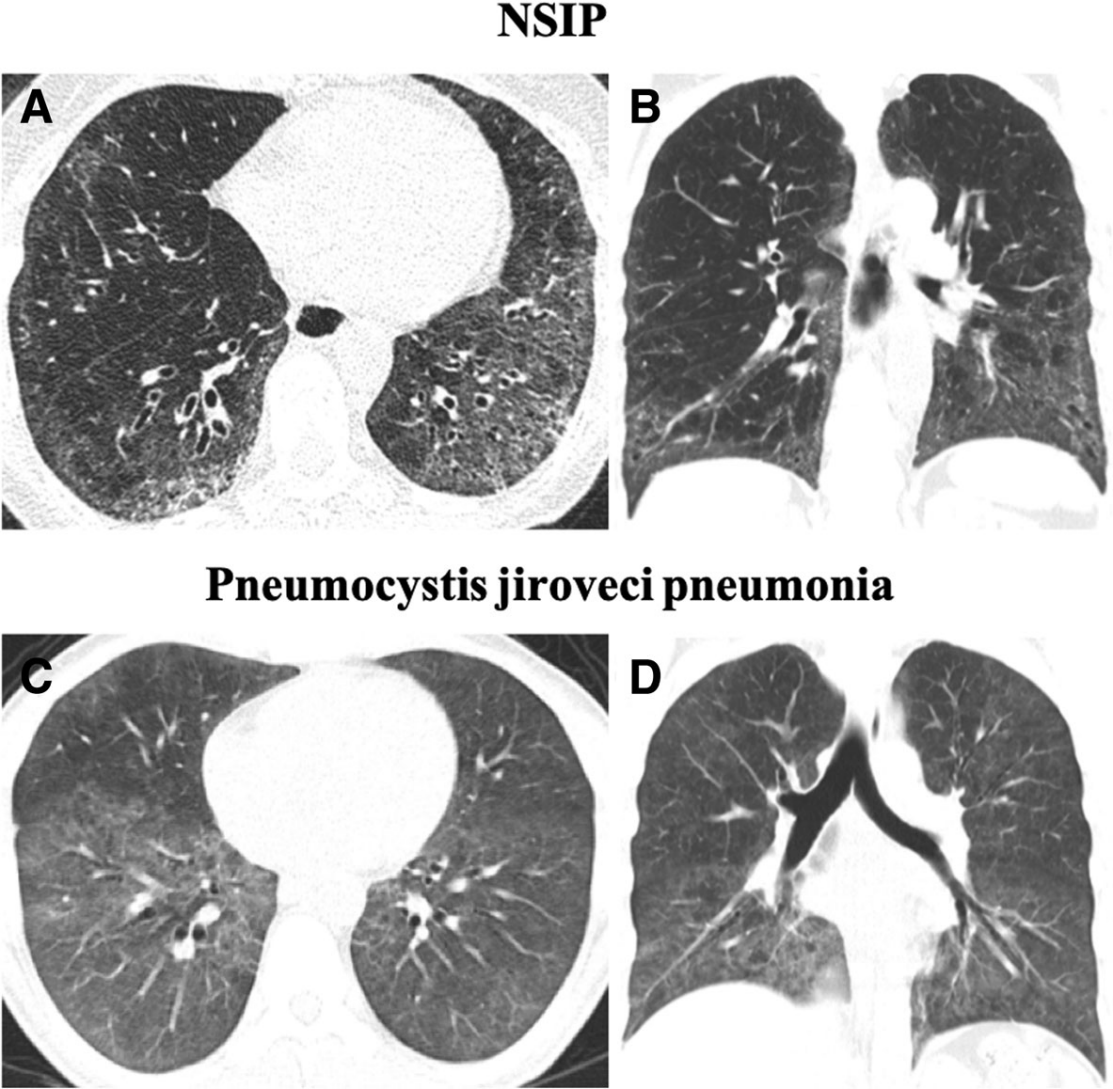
Differential diagnosis of DIP. a Axial and b coronal CT images depict NSIP in a female patient with systemic sclerosis. CT findings show bilateral and asymmetrical ground-glass opacities, with a lower lobe predominance and immediate subpleural sparing. c Axial and d coronal CT images depict pneumocystosis in an immunocompromised patient with HIV. Imaging findings show bilateral ground-glass opacities, predominantly in the lower lobes
